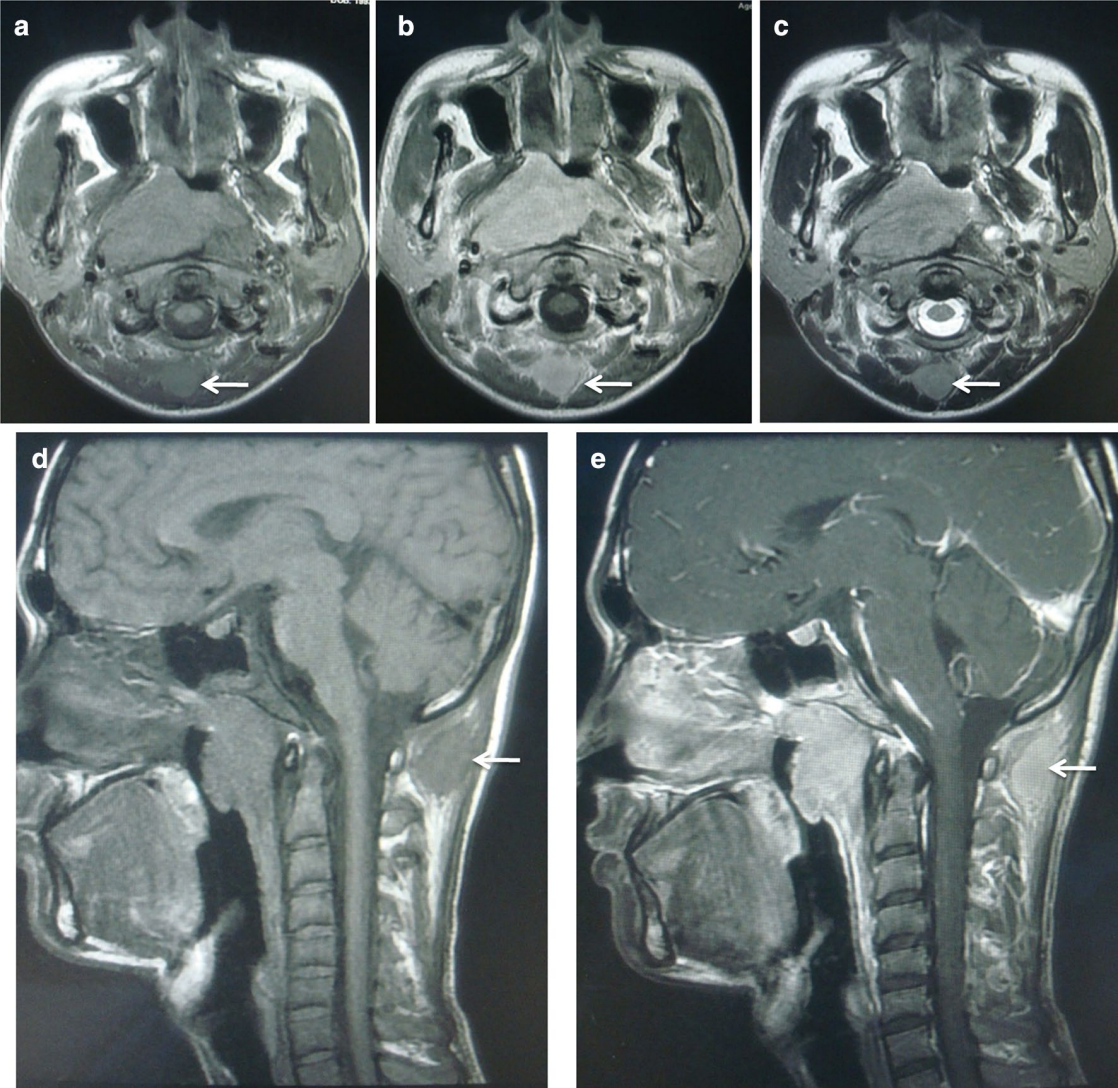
Magnetic resonance (MR) imaging of the NPC patient before treatment. T1-weighted axial MR images a without contrast, b with contrast, and c T2-weighted axial MR image show an occipital lymph node (18 mm × 19 mm) with equal T1 signal, long or equal T2 signal, and obvious enhancement (*arrows*). T1-weighted sagittal MR image d without contrast and e with contrast also show an enlarged lymph node with enhancement in subcutaneous tissue of the occiput (*arrows*)
